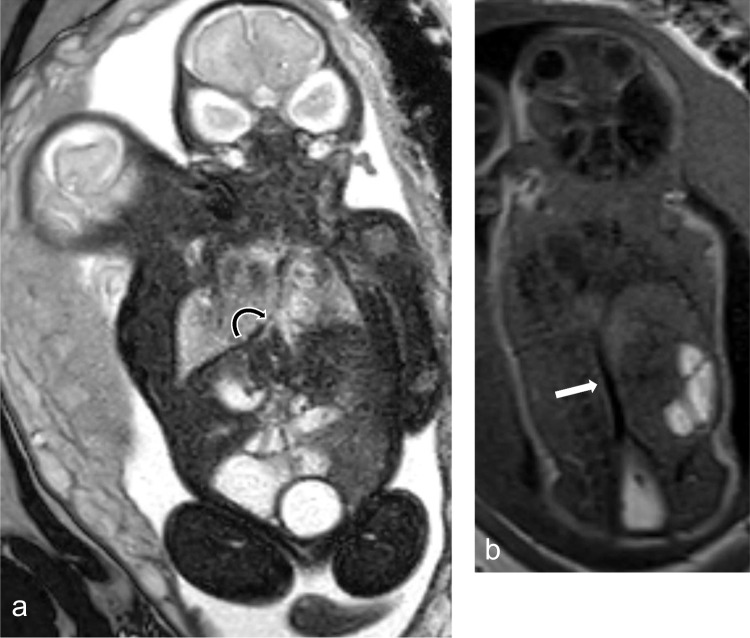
Fetal MRI of dicephalic parapagus at gestational age 32 weeks, 1 day (same case as depicted in Fig. 6), showing vascular sharing. a BTFE image coronal to the conjoined torso shows the hepatic veins of the shared liver draining into the heart of the left-sided twin (*curved black arrow*). b T1-weighted image coronal to the conjoined torso shows a flow void in the shared abdominal aorta (*white arrow*), which appeared to be a continuation of the left-sided twin’s thoracic aorta. *BTFE*, balanced turbo-field echo
